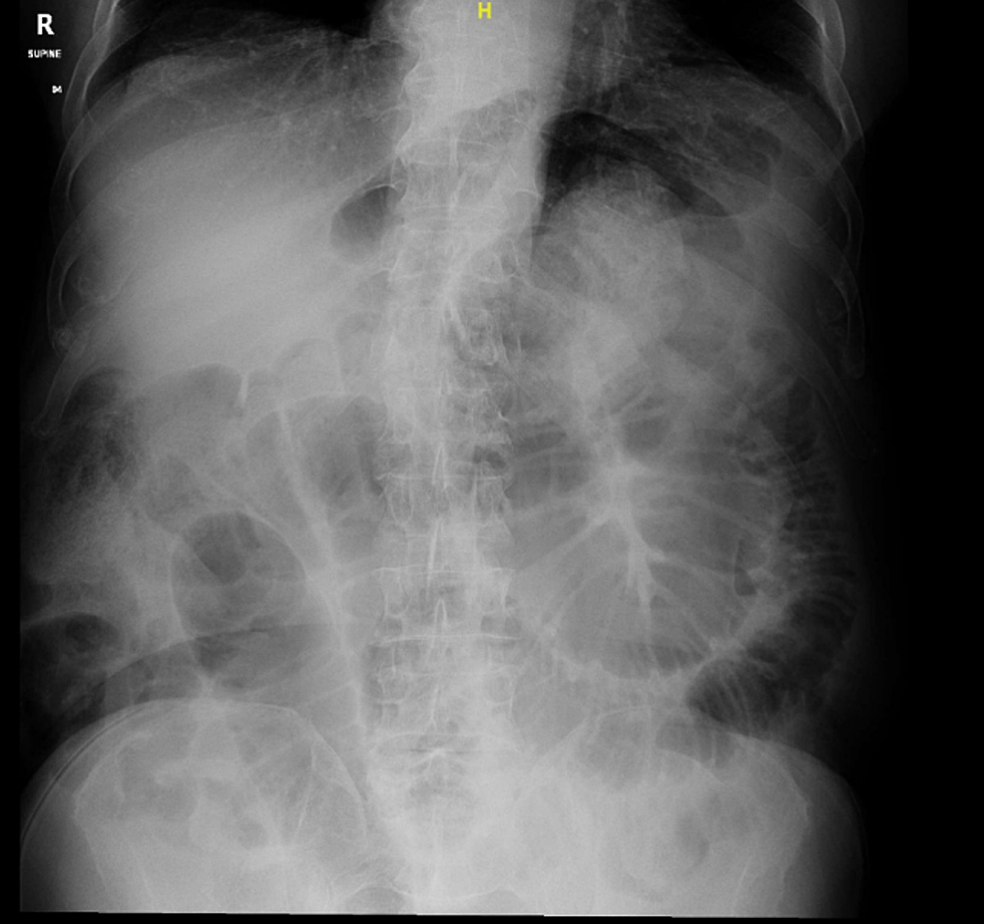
Abdomen X-ray showing dilated small bowel loops
